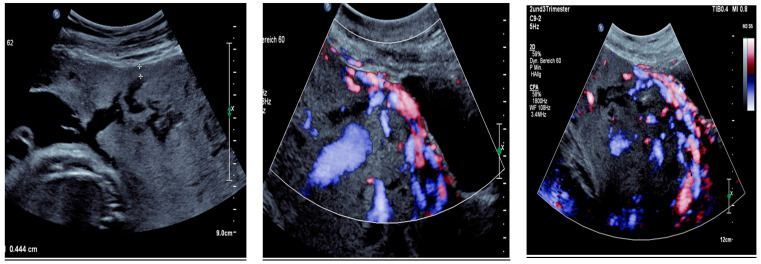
Revealed the following sonographic findings:
